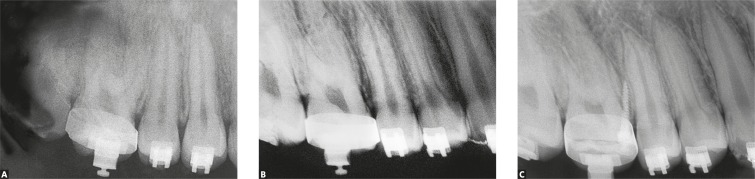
Periapical radiographs. A) Reduced space between #15 and 16 roots; B)
